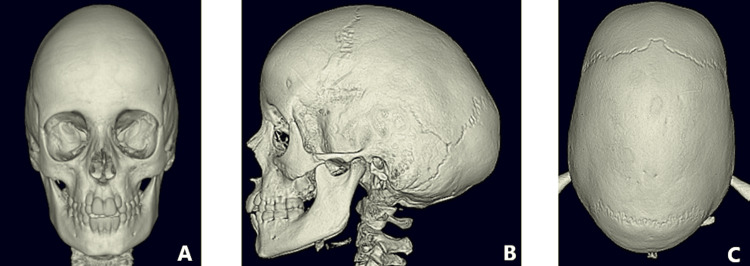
Pre-operation CT scans (A) Pre-operation anterior-posterior view CT; (B) Pre-operation lateral view CT; (C) Pre-operation vertex view CT
